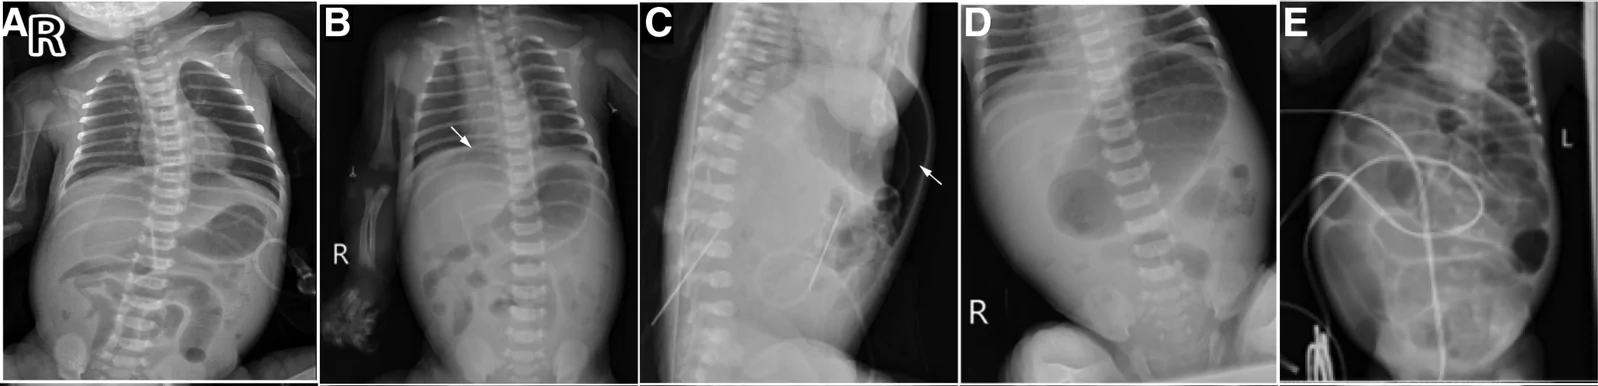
Representative abdominal radiographic findings in a term-born small-for-gestational-age infant with neonatal gastrointestinal perforation. (A) Pneumatosis intestinalis and segmental gas accumulation in the intestinal tract; (B) anteroposterior view of pneumoperitoneum; (C) lateral view of pneumoperitoneum; (D) anteroposterior view; and (E) intestinal dilation.
